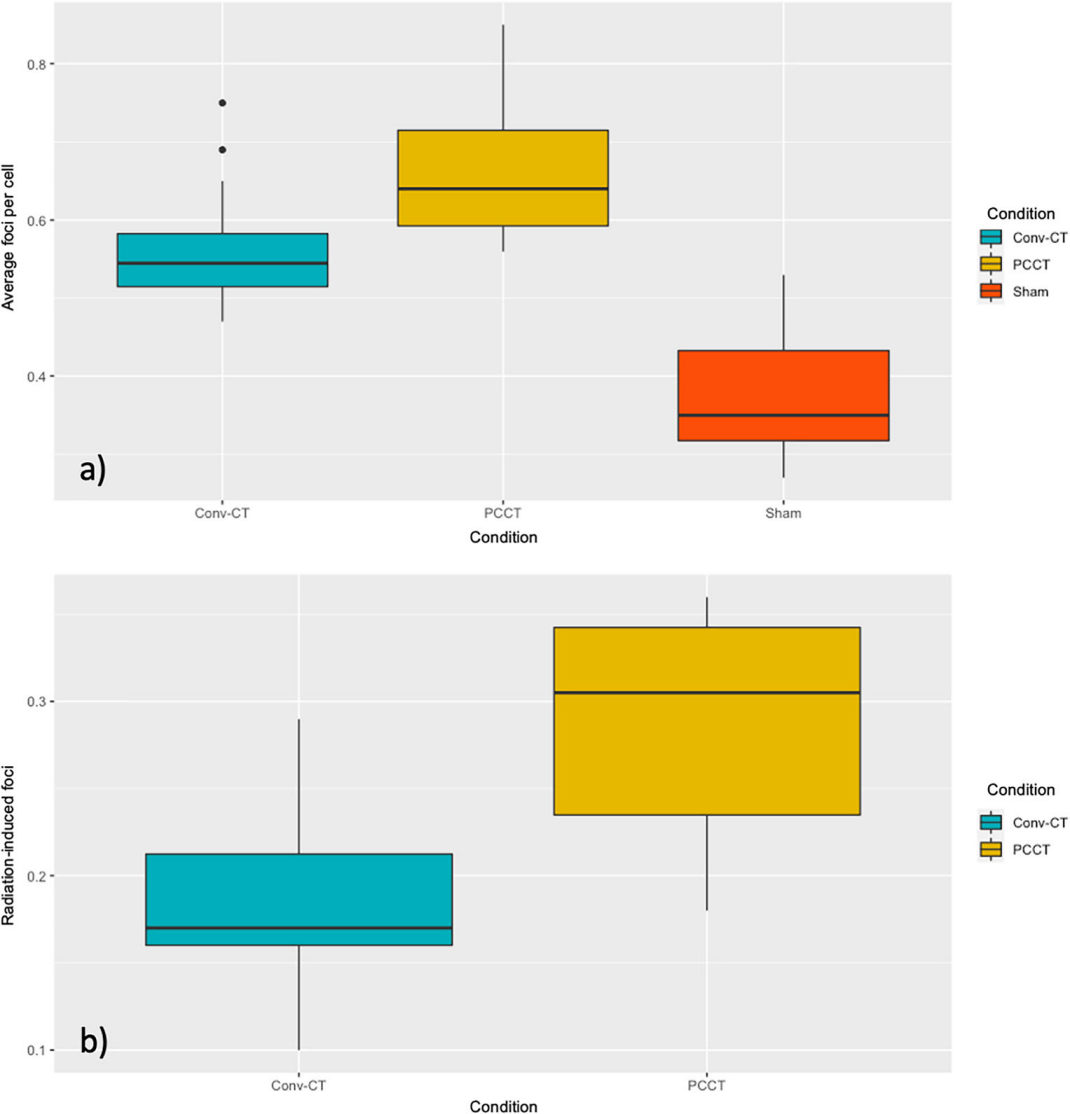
Number of average DNA double-strand break foci per cell (a) and radiation-induced foci (b) counted after photon-counting CT (PCCT), conventional CT (Conv-CT), and sham exposure (Sham). Boxes display the interquartile range with the median as a horizontal line and whiskers for minimum and maximum. PCCT resulted in significantly elevated average foci numbers per cell (*p* < 0.004) as well as radiation-induced foci (*p* < 0.0001) compared to conventional CT
